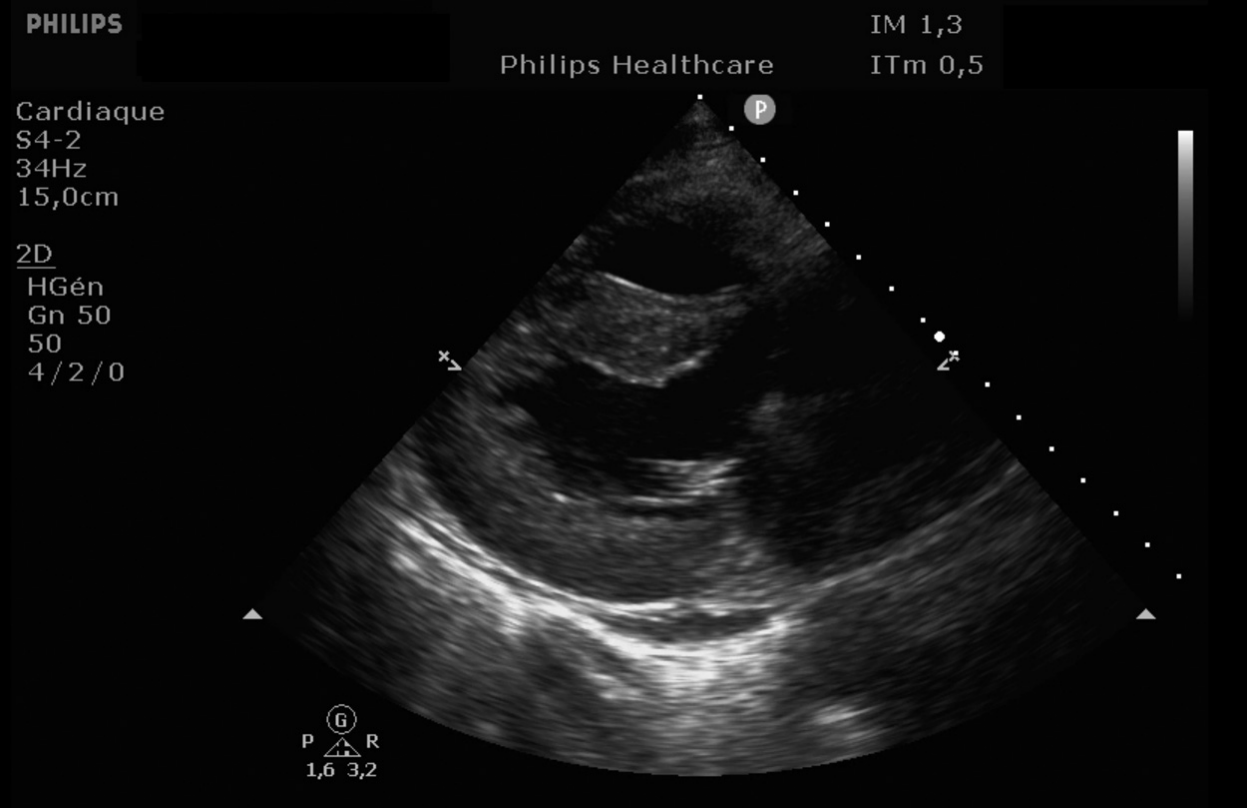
Parasternal long axis of the heart
